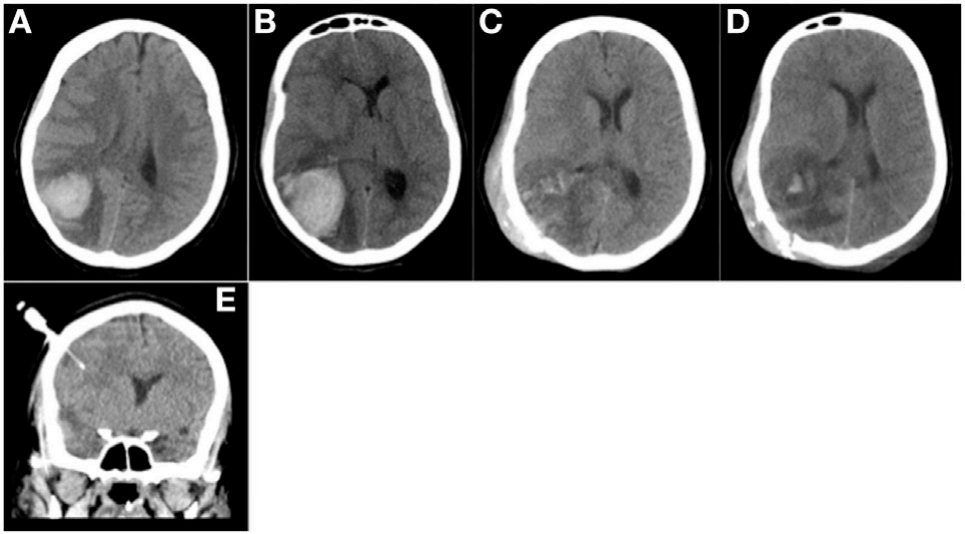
Patient 2. CT head (A,B) prior to surgery: (A) Day 1: 36 mL parieto-occipital hematoma with perilesional edema and slight mass effect. (B) Day 3: significant hematoma expansion (hematoma volume measured at 50 mL) and increased mass effect. CT head (C–E) after surgery: (C) Day 5; the day after surgery: hematoma evacuation; decreased mass effect; subcutaneous hematoma occurring during surgery. (D) Day 10: no rebleeding was noted. (E) Day 10: no hemorrhage was observed around the intraparenchymal probe (intracranial pressure monitoring).
